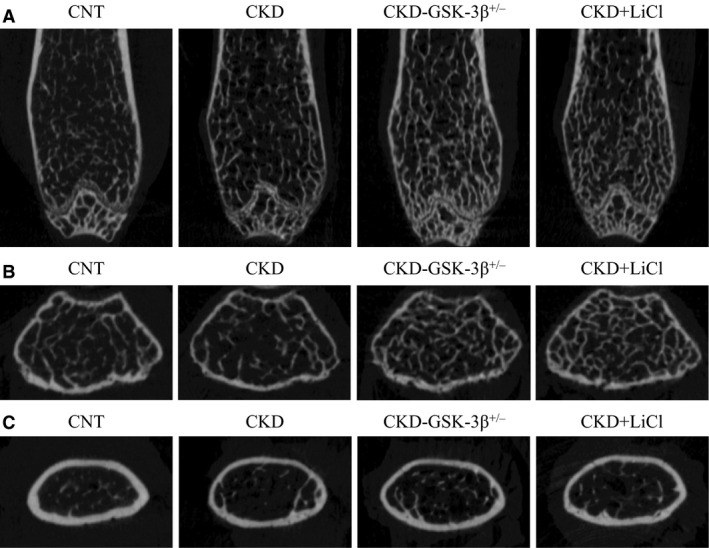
Representative microcomputed tomography images of femurs. (A) Longitudinal images of femurs. (B) Cross‐sectional images of femurs in trabecular bone area. (C) Cross‐sectional images of femurs in cortical bone area. CKD, chronic kidney disease; CNT, control; GSK‐3*β*
